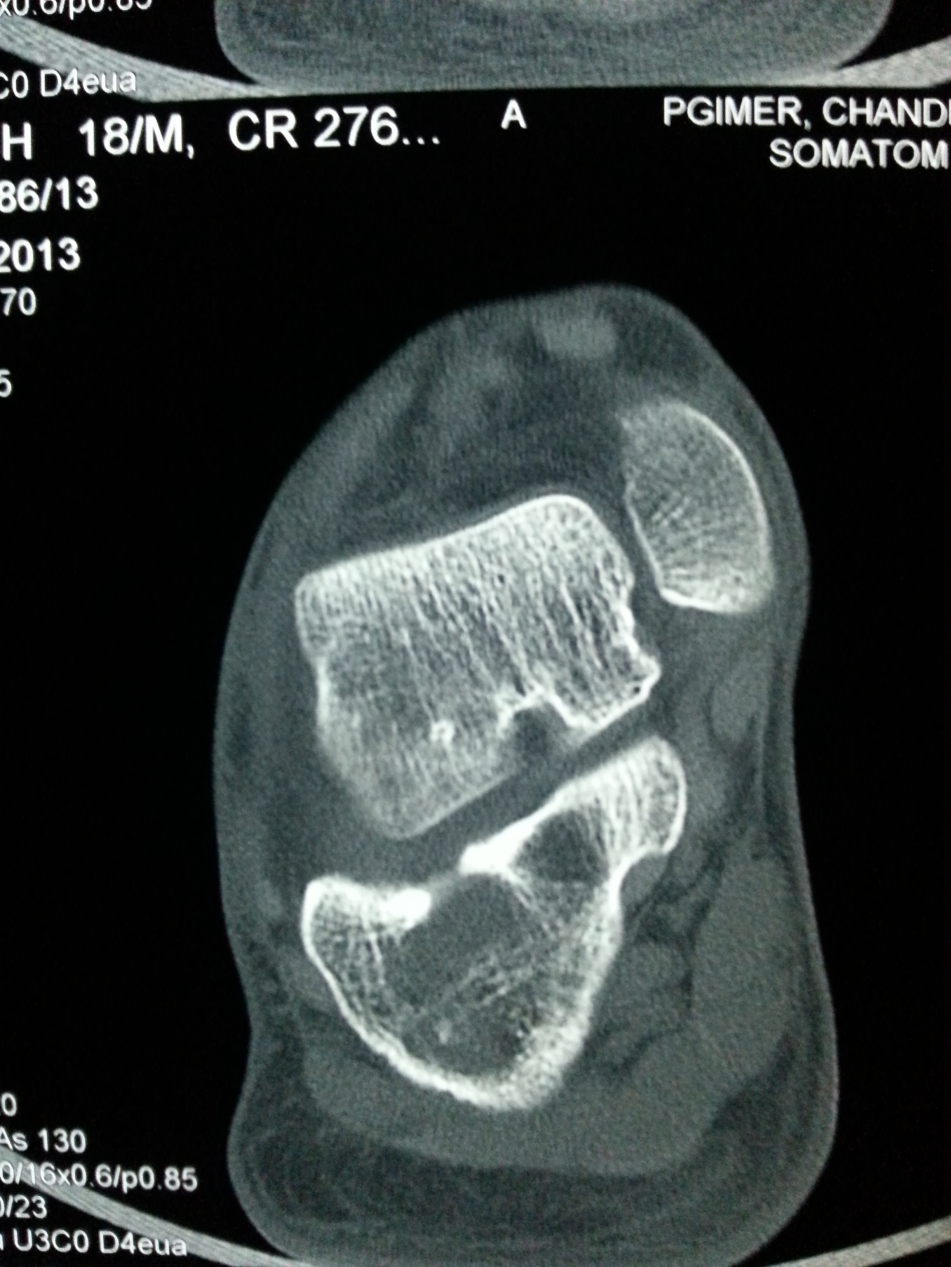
CT scan showing extensile destructive lesion in same case.
